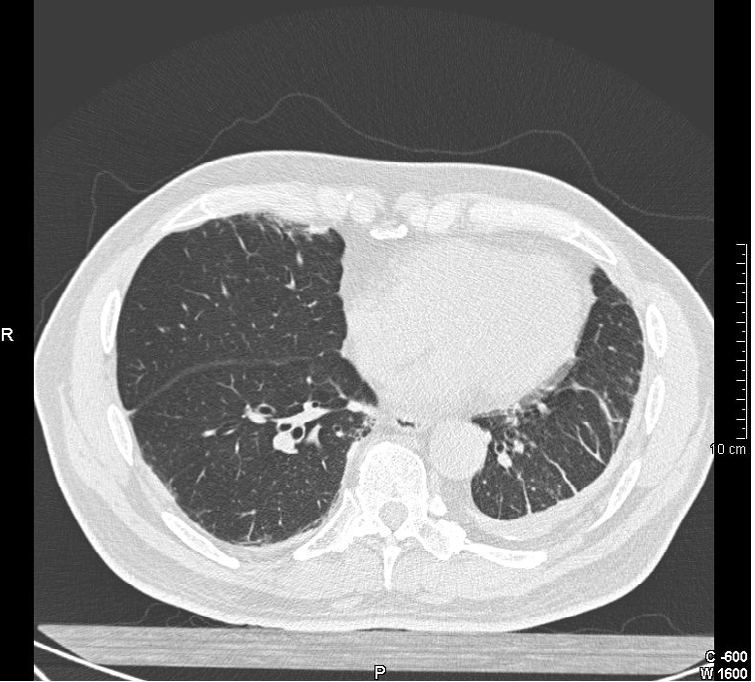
Computed tomography (CT) scan of the thorax demonstrating asbestos-related diffuse pleural thickening. Note the "crow's feet" or parenchymal bands which are clearly seen on the left, and the overall reduction in lung volume.
